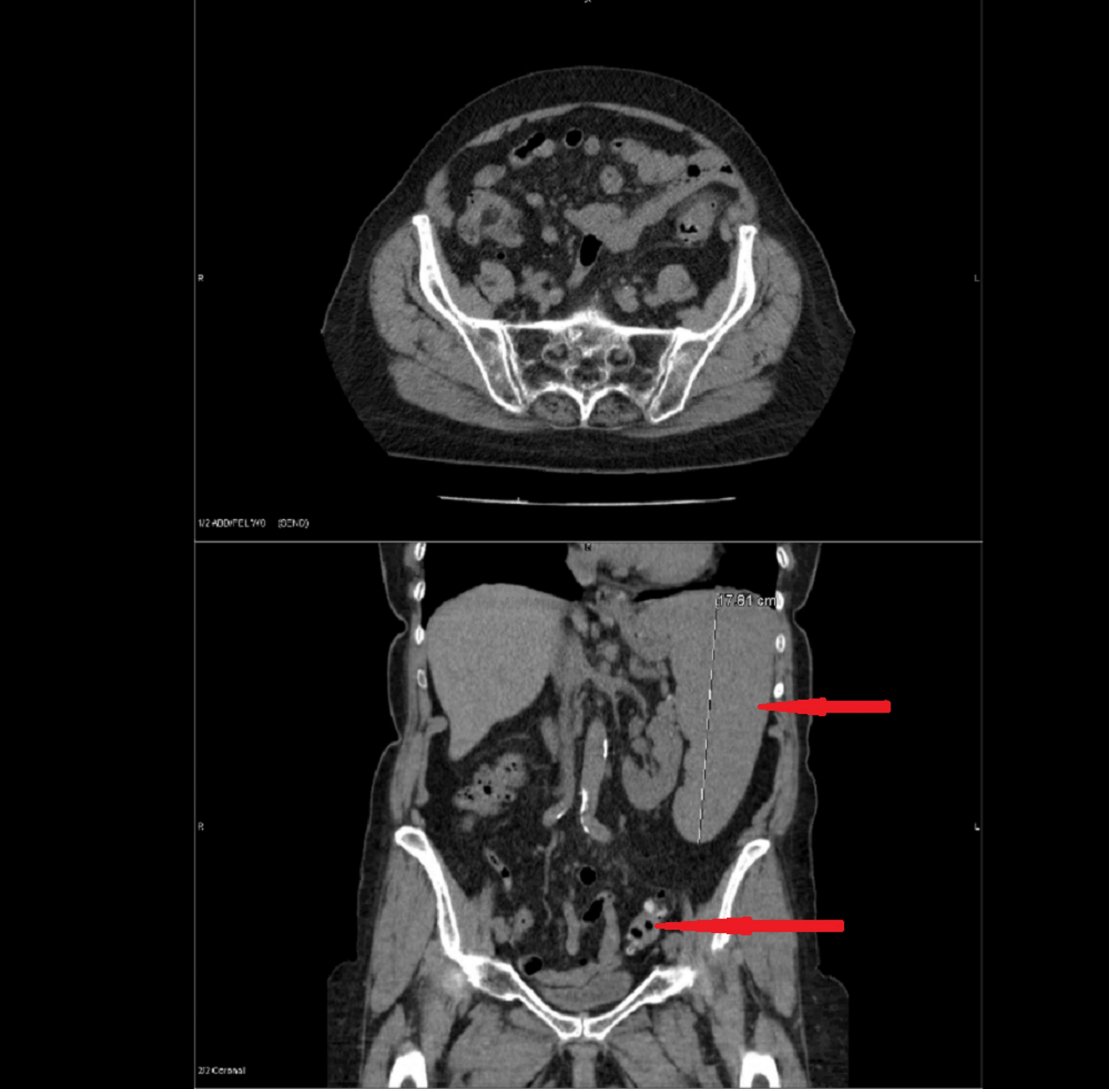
Computed tomography scan abdomen and pelvis with intravenous contrast showed Splenomegaly (Red arrow 1) and Sigmoid diverticulitis (Red arrow 2)
